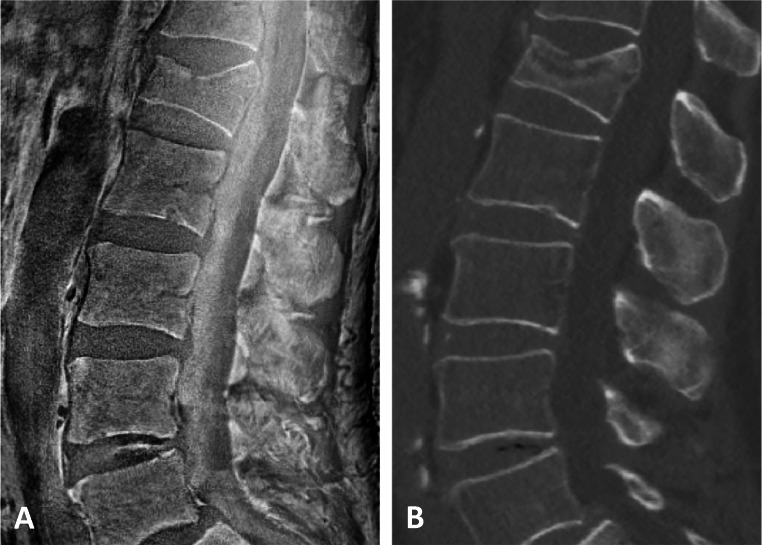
UTE-derived SW-like images with CT-like contrast of an acute compression fracture of the 1st lumbar vertebra (A). The fracture can be evaluated equivalent compared to the conventional CT scan (B)
